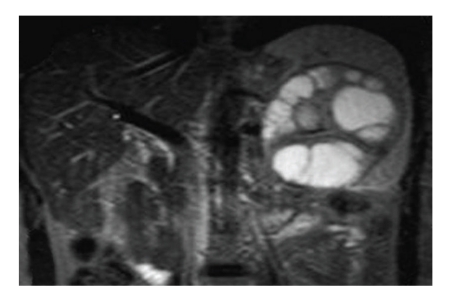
Coronal T2-weighted MRI shows multivesicular cystic lesion located in the middle and lower pole of the spleen, containing hypointense rim and septae.
